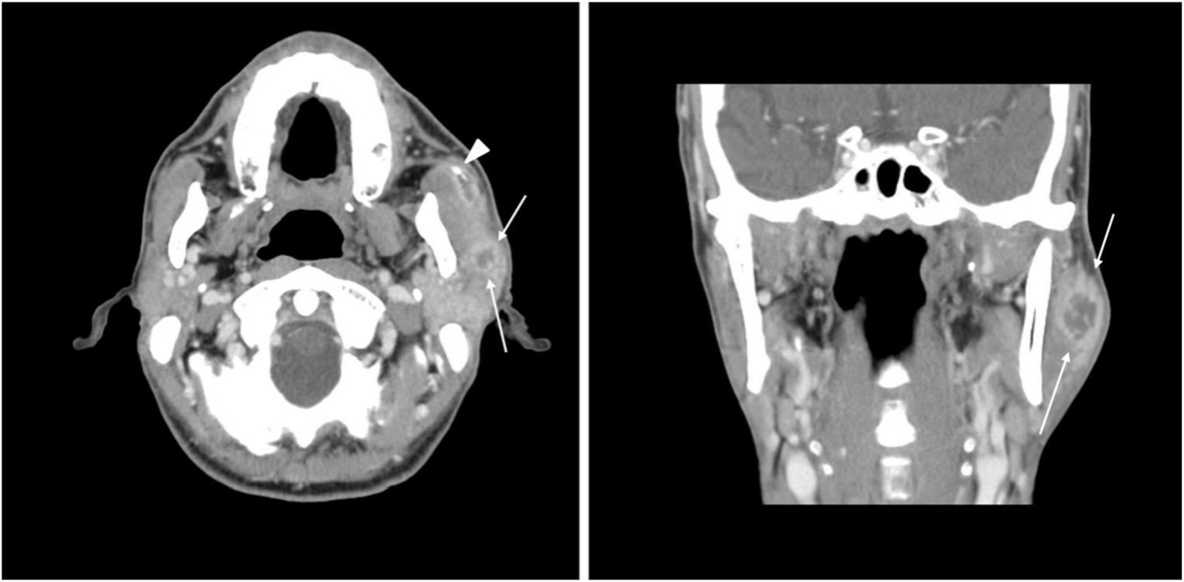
Pre-operative mandible CT of the case 2 patient on first visit. Mandible CT showing salivary stone (*arrow head*) and acute/chronic suppurative sialoadenitis with irregular thick-walled, septated cystic mass (*arrow*) in superficial lobe of right parotid gland
